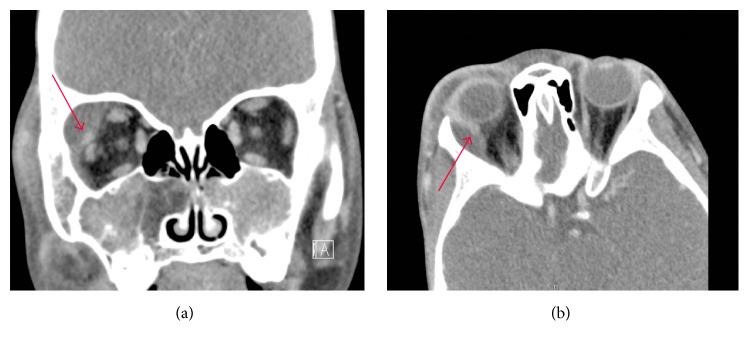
Computed tomography (CT) images. (a) Coronal CT slice exhibiting fluid collection with intraorbital mass effect (arrow). (b) Axial CT slice showing extensive soft tissue edema (arrow).
